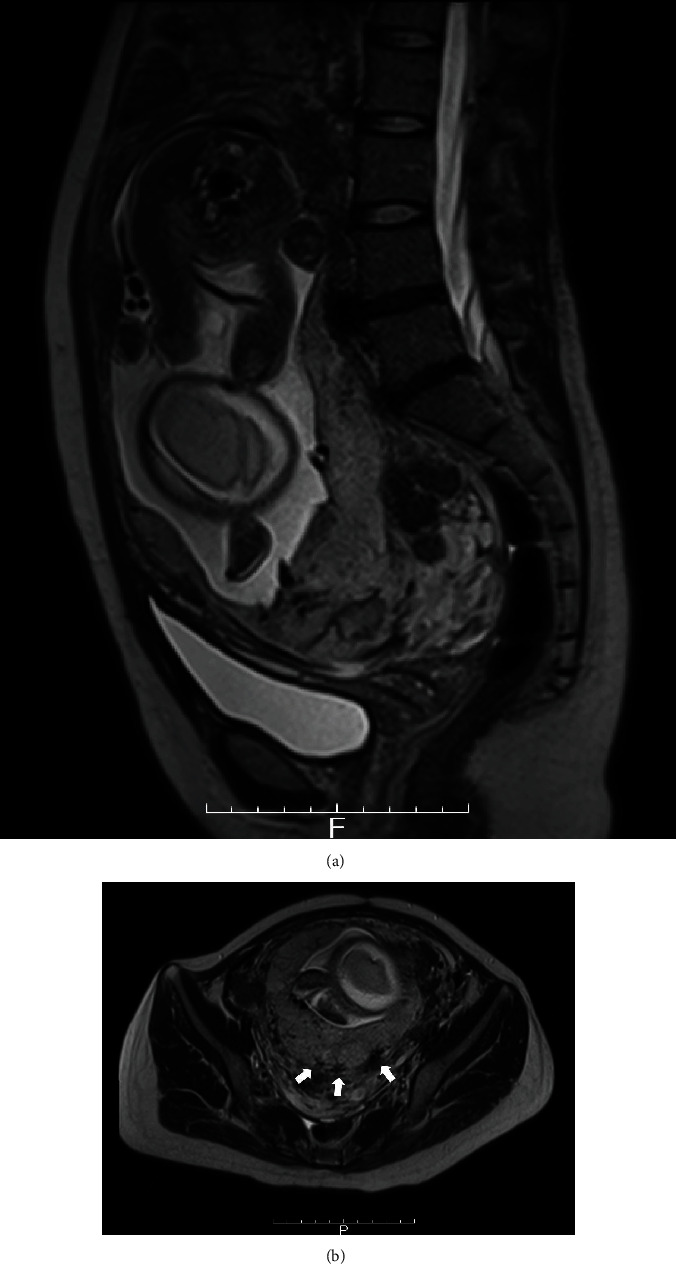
MRI findings of placenta previa. (a) Sagittal and (b) axial image. Arrows represent placental recess (a wedge-shaped contraction of the placental surface and uterine outer rim accompanying a T2 dark band).
